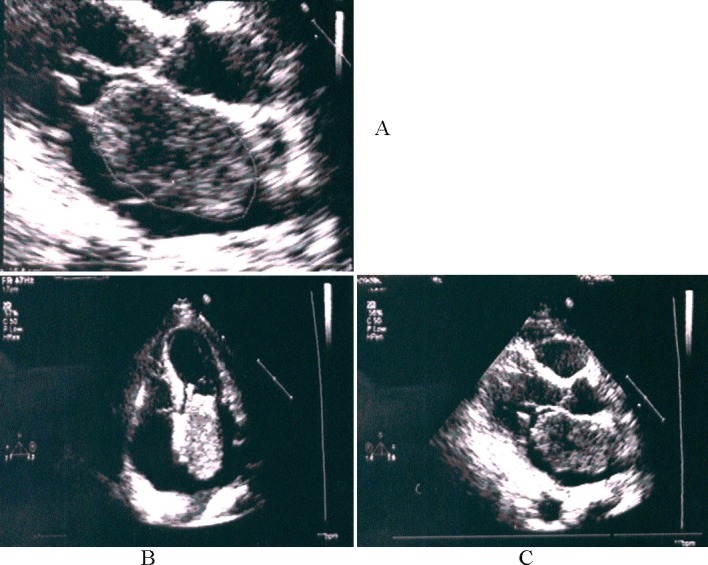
A: show a large mass 16 cm^2^ with attachment to the atrial septum; B: there is also a diastolic protrusion with obstruction towards left Long axis apical view during diastole; C: apical four chamber view during systole.
